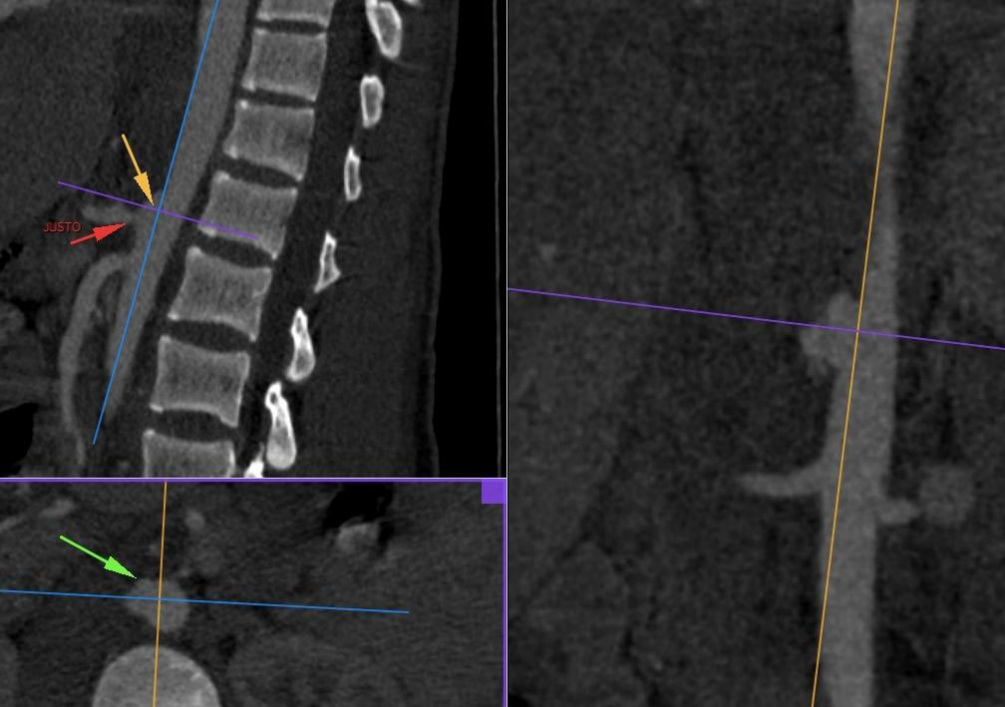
Computed tomography angiography images showing an aortic pseudoaneurysm at the thoracoabdominal transition, adjacent to the origin of the celiac trunk.
